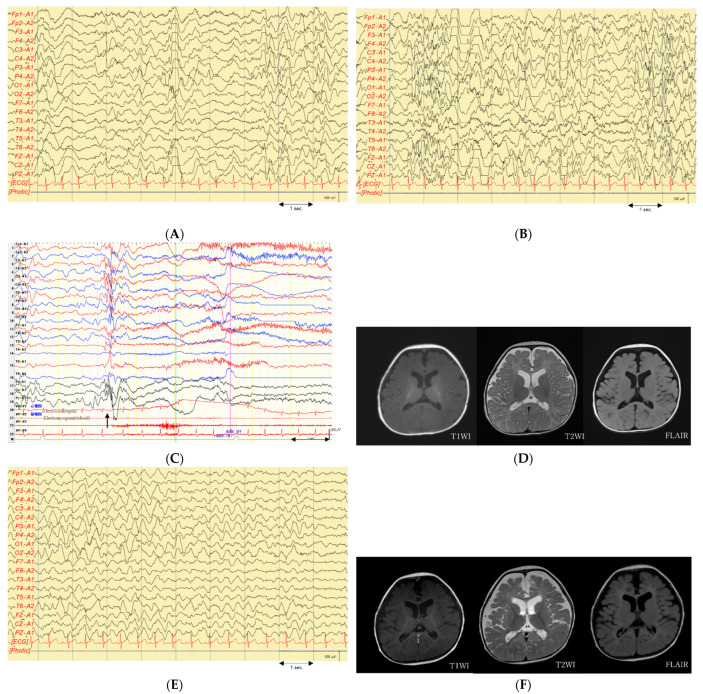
EEGs and brain MRIs of the patient. (A) Interictal EEG at 2 months of age showing localized spike waves and spike–slow wave complexes in the right frontal, central, and occipital regions, which further broadened. Additionally, a left central spike wave was observed; (B) Interictal EEG at 3 months of age showing frequent irregular high-amplitude spikes and slow waves without left–right synchrony during sleep and wakefulness, indicative of hypsarrhythmia; (C) Ictal EEG at 3 months of age revealing spasms coinciding with irregular poly-spike waves followed by high-amplitude slow-wave complexes (arrowed area) and desynchronization; (D) Brain MRI at 3 months of age shows no obvious structural abnormalities that could cause seizures; (E) Interictal EEG at 11 months of age revealing slow waves at 3–4 Hz that were continuously observed on both sides as background waves without hypsarrhythmia; (F) Brain MRI at 1.4 years of age showing diffuse cerebral atrophy and corpus callosum thinning; however, there were no obvious abnormal structures.
